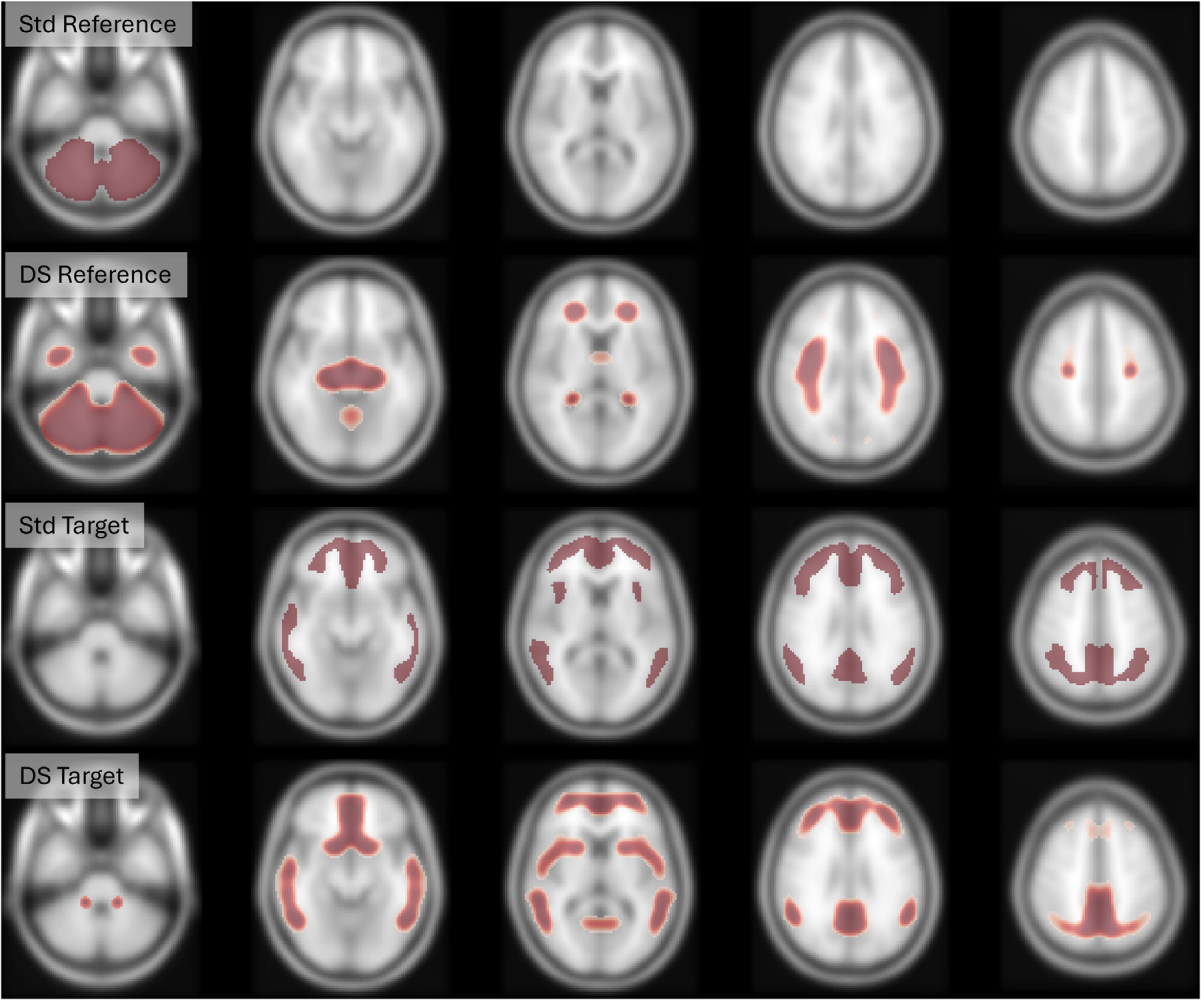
Axial views of the Standard CL reference mask (row1) and the new reference mask derived from DeepSUVR (row2), the Standard CL target mask (row3), and the new target mask derived from DeepSUVR (row4).
